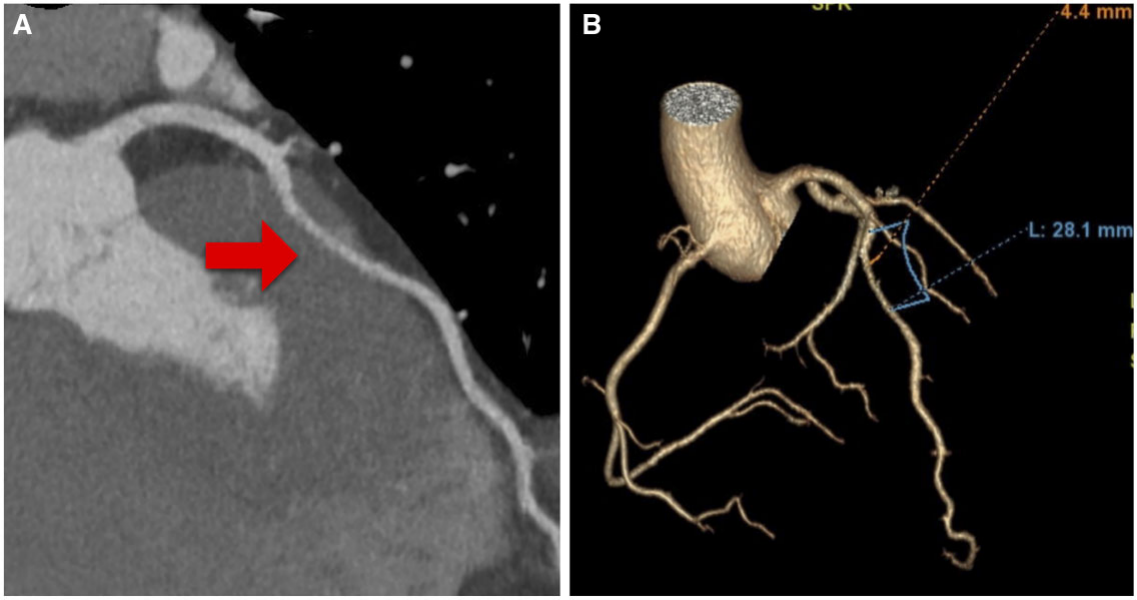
(A) Coronary computed tomographic angiography showing a myocardial bridge of the mid-left anterior descending artery (arrow), length 28.1 mm and depth 4.4 mm. (B) Three-dimensional reconstruction.
